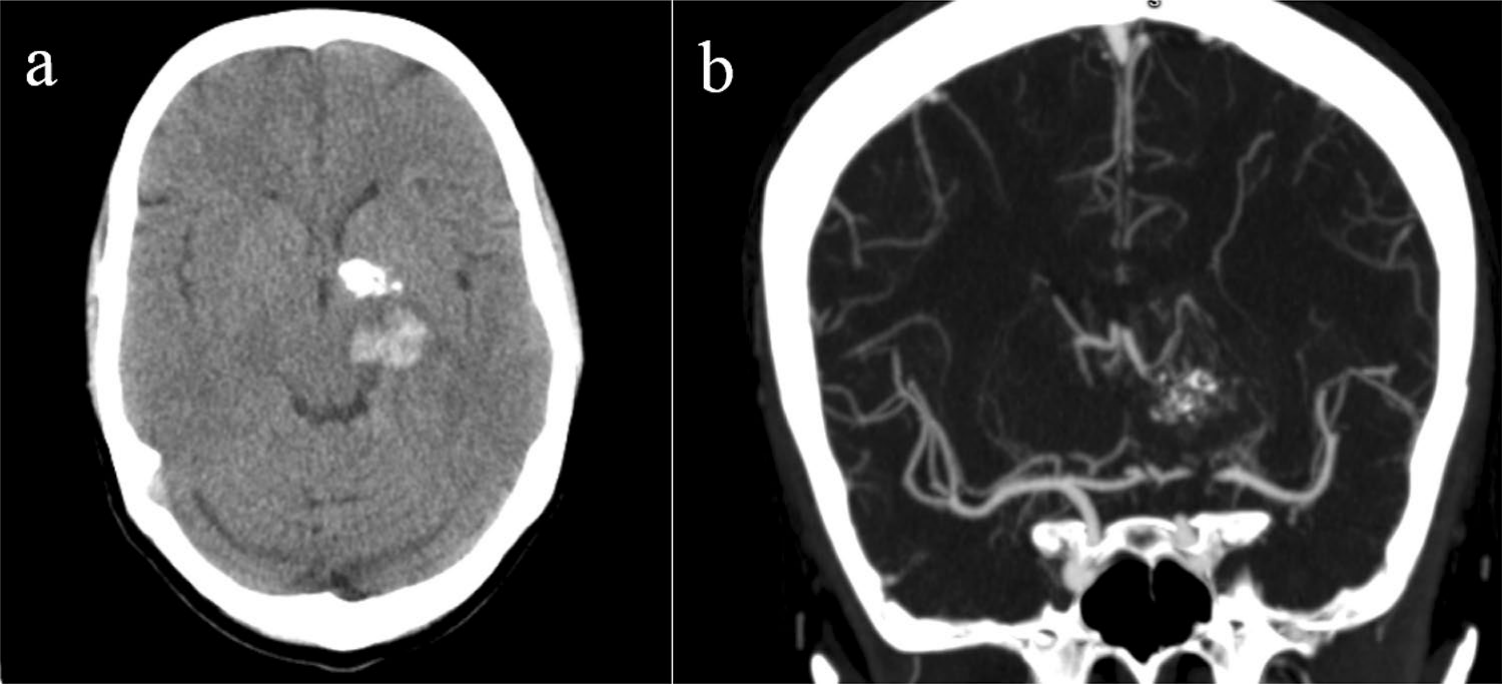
Axial non contrast CT scan (a) shows left basal ganglia hemorrhage and a round calcified lesion near it. The findings are high probable of underlying vascular lesion and showed to be an Arteriovenous malformation (AVM) in brain CTA (b).
